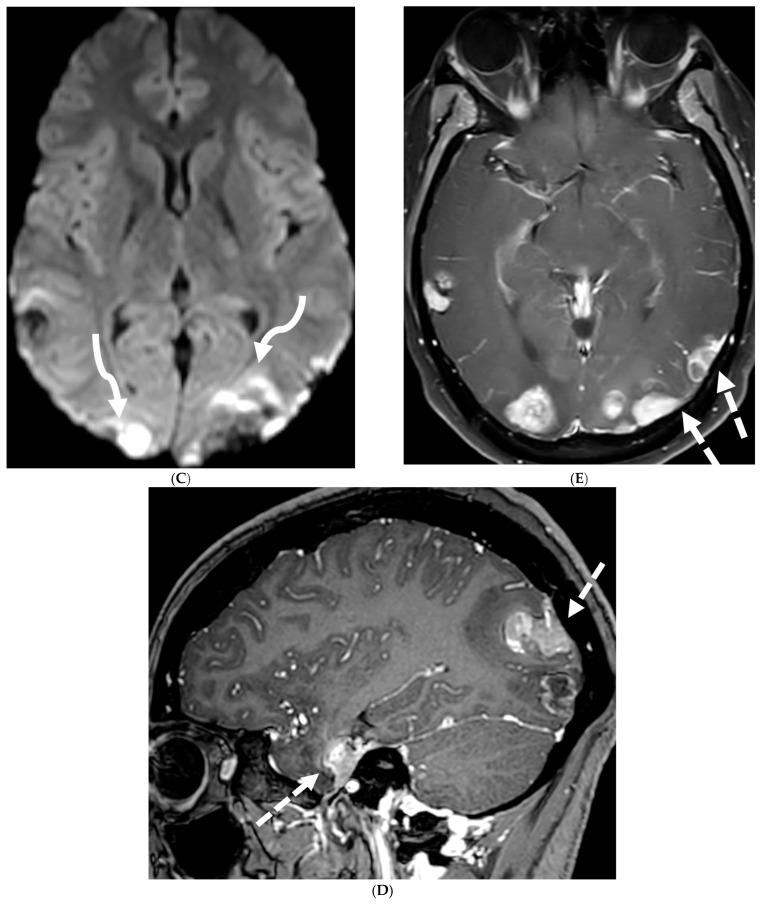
Axial T2 FS (A), axial Susceptibility weighted imaging (SWI) (B), axial DWI (C), post contrast sagittal and axial T1 post contrast (D,E): 19-year-old female with history of stage IV neuroblastoma, left paraspinal primary ganglioneuroblastoma, treated with chemotherapy, radiation and bone marrow transplant presents with headache. There are extensive hemorrhagic leptomeningeal masses, both supra and infratentorial region (arrows). The lesions also demonstrate restricted diffusion which could be secondary to internal hemorrhage or high cellularity of the tumor(curved arrows). The larger masses invade the cortex of both cerebral hemispheres, with surrounding vasogenic edema (black arrow). Avid enhancement of the lesions is seen along with overlying dura (dashed arrows). Features are in keeping with extensive leptomeningeal metastatic neuroblastoma.
